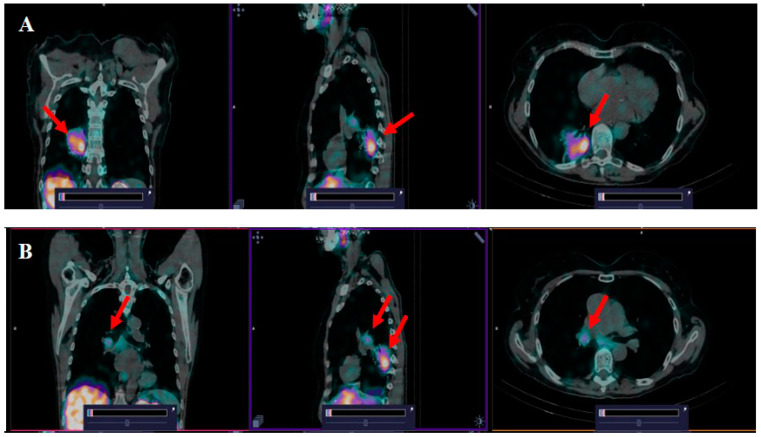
SPECT/CT images with [^99m^Tc]Tc(CO)_3_-(HE)_3_-Ec1 in patient 9 with cancer of the lower lobe of the right lung and regional lymph nodes metastases 2 h after injection. The upper setting of the SPECT scale window 5.66% of the maximum number. (A) The accumulation of [^99m^Tc]Tc(CO)_3_-(HE)_3_-Ec1 in the projection of the primary lung tumor (SUV_max_ = 6.8) (red arrows). (B) The accumulation of [^99m^Tc]Tc(CO)_3_-(HE)_3_-Ec1 in paratracheal lymph nodes affected by metastases (SUV_max_ = 2.47) (red arrows).
